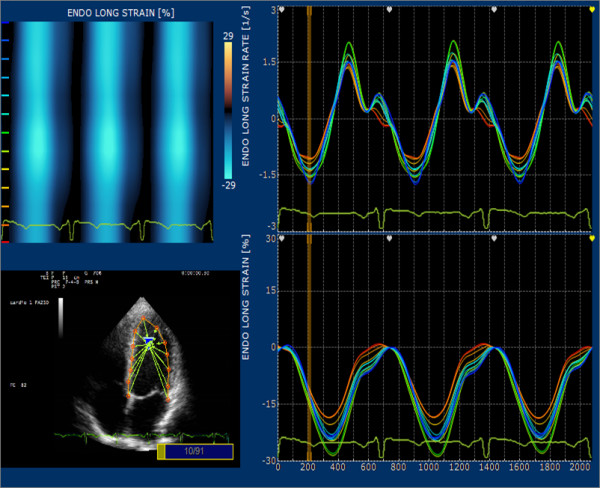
Graph with parts of the processing result of an apical four chambers view. The vectors show the direction of displacement of any point selected on top left, on upper left color scale analysis of systolic strain, on the right side curves for Strain and SR any selected points.
